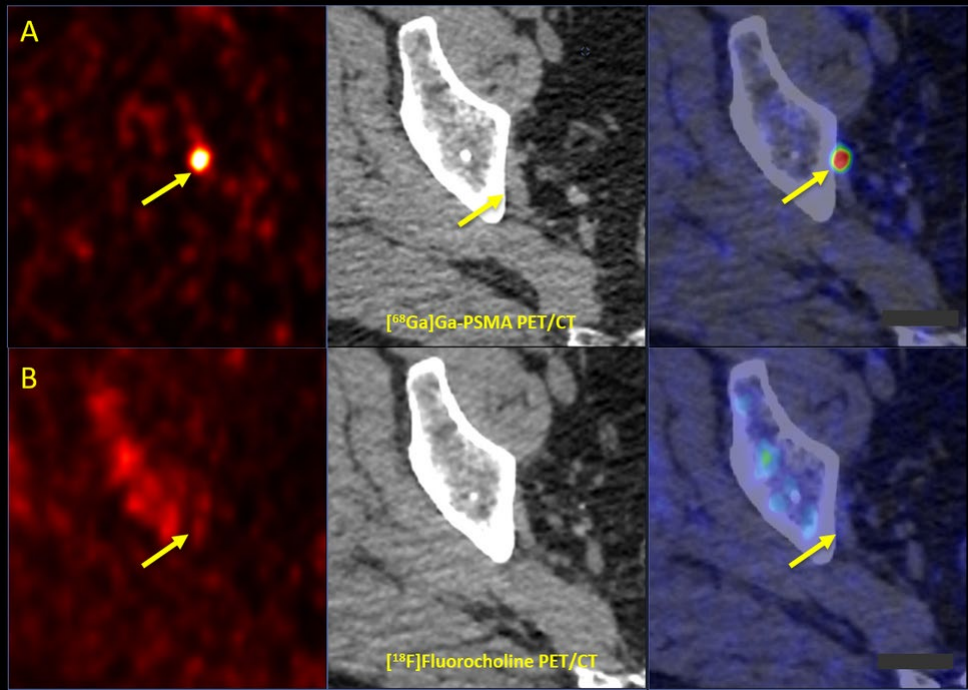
A [^68^Ga]Ga-PSMA and B [^18^F]Fluorocholine PET/CT in a prostate cancer patient with biochemical recurrence (Gleason score 3 + 4, tPSA: 1.85 ng/ml, PSAdt: 12 months) after RPE, RTx and ADT. [^68^Ga]Ga-PSMA (upper row) PET/CT (transaxial PET (left), CT (middle), and fusion PET/CT (right) images) shows focal uptake (SUV max = 16.2) in a right external iliac lymph node (diameter: 6 mm, arrows) suggesting lymph node metastasis. No [^18^F]Fluorocholine (lower row) uptake. *RPE* Radical Prostatectomy, *RTx* Raditherapy, *ADT* Androgen Deprivation Therapy, *tPSA* Trigger Prostate Specific Antigen, *SUV max* Maximum Standardized Uptake Value, *PSMA* Prostate-Specific Membrane Antigen, *PET/CT* Positron Emission Tomography/Computed Tomography
